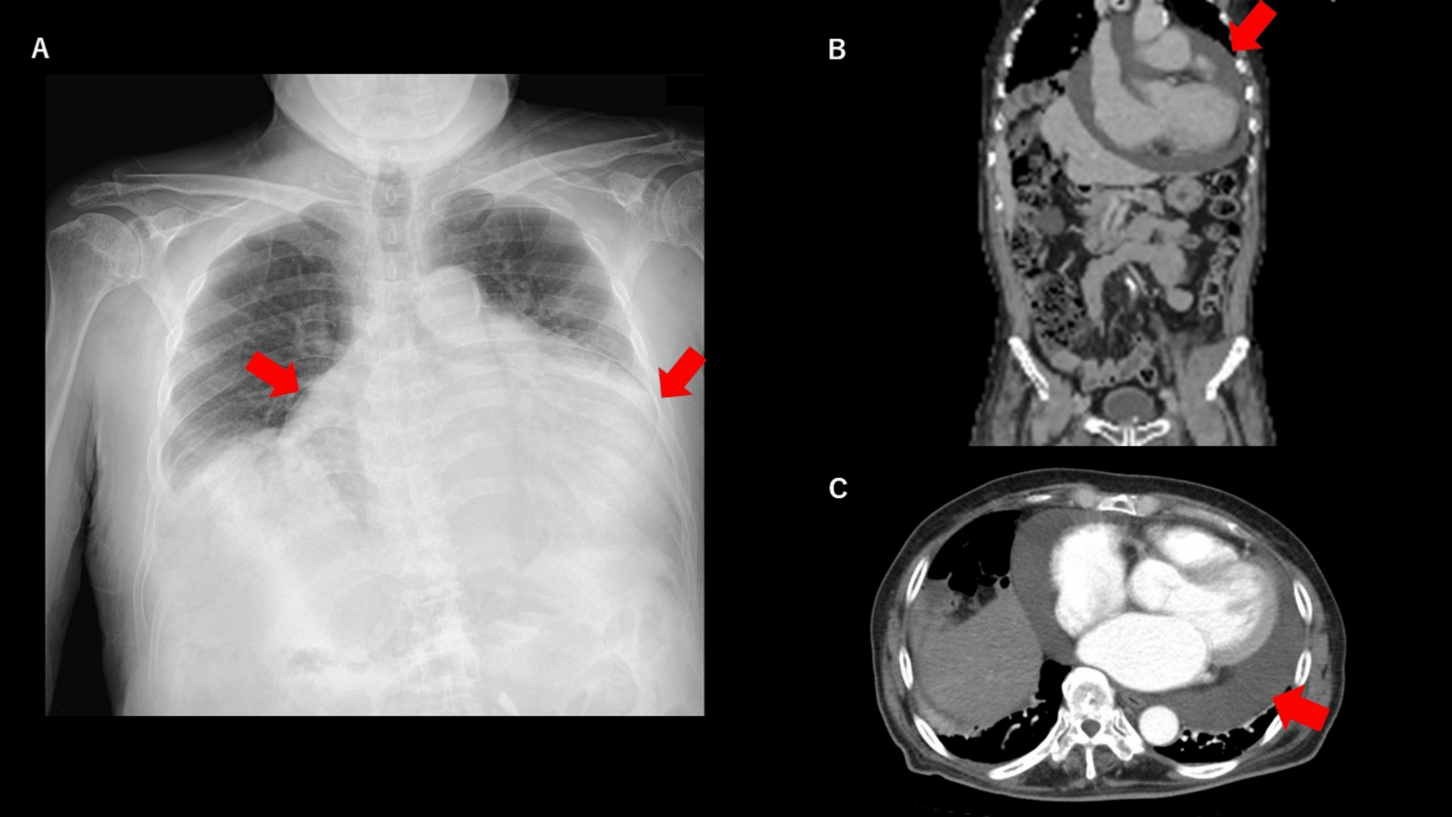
Chest X-ray and CT scan before surgery. A) chest X-ray before surgery. Significant cardiomegaly is shown with red arrows. B) sagittal view of contrast body CT scan. Pericardial effusion is remarkable (red arrow). C: axial view of contrast chest CT scan. Significant cardiomegaly and pericardial effusion are shown (red arrow).
